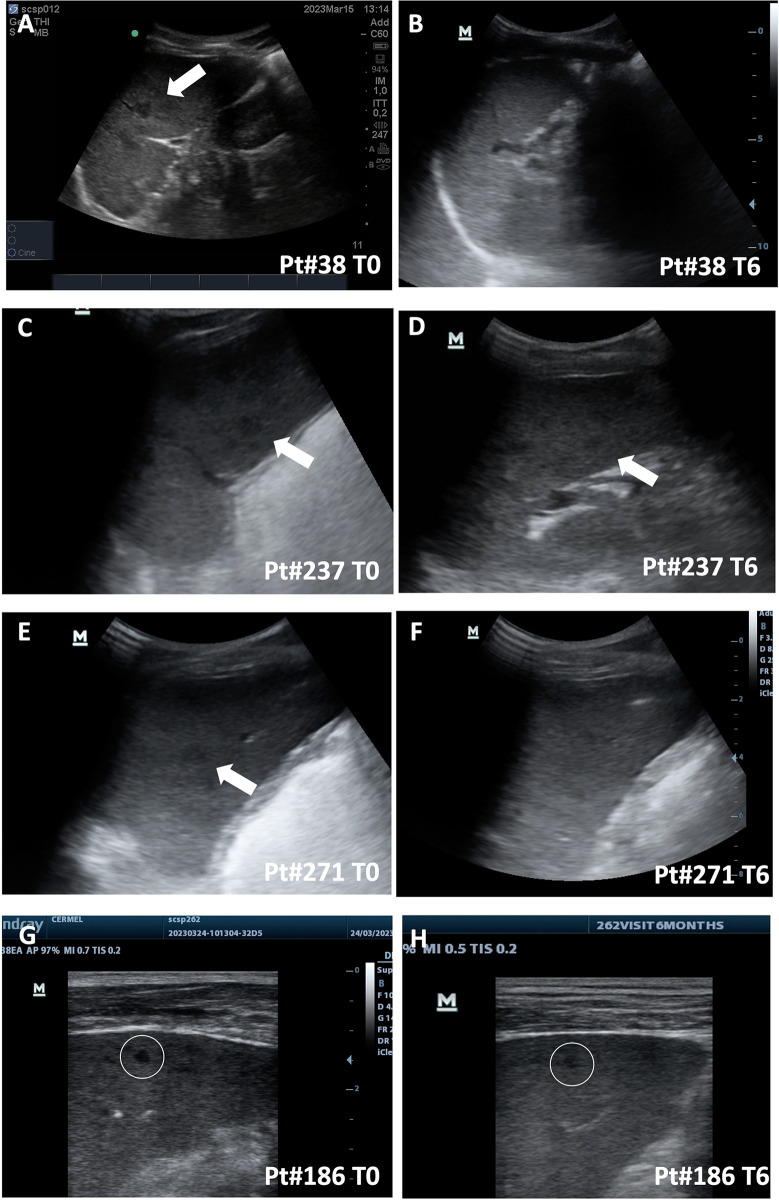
Representative static ultrasound views of focal spleen lesions at diagnosis (T0) and 6 months follow-up (T6). Videos of the ultrasound exams are available as S1–S14 Videos, to allow a better and comprehensive visualization of the lesions in the whole organ. Participant (Pt) identification code (Pt#n) corresponds to the code available at https://zenodo.org/records/13169677. Macro nodules shown in the static section are indicated by a white arrow; micronodules by a white circle A, C, E] participants with macronodules at T0, convex probe. B, D, F] 6 months follow-up ultrasound of participants with macronodules at T0, convex probe; note that modules are virtually disappeared in Pt#38 and Pt#271, while they are still visible in Pt#237. G, H] Representative T0 (G) and T6 (H) ultrasound of participant with micronodules, only visible with linear probe.
